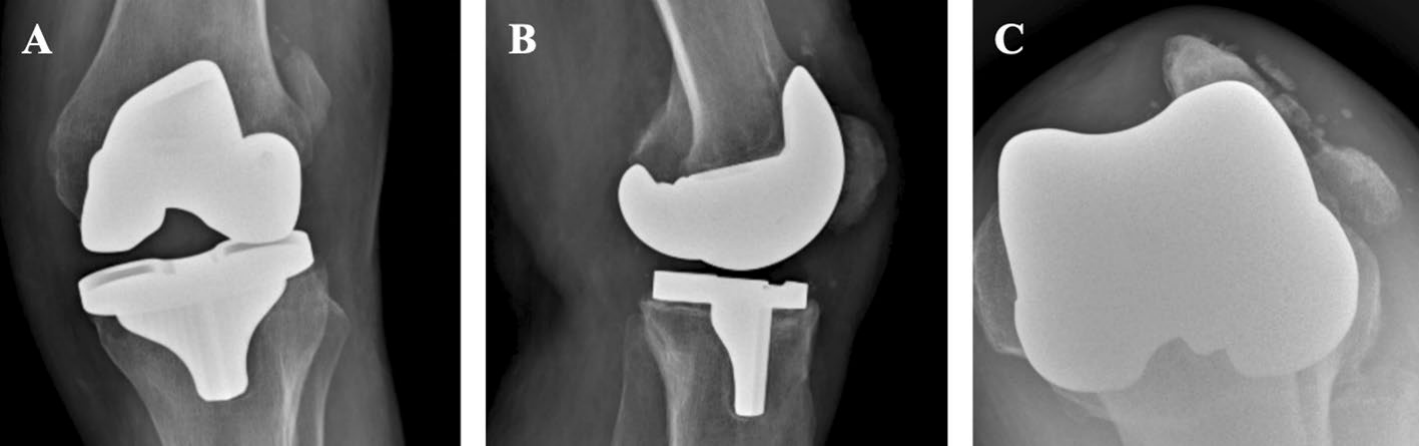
Periprosthetic patellar fracture in the non-resurfaced setting with failure of the extensor apparatus in a left knee. A Anteroposterior X-ray view; B Lateral X-ray view; C Merchant X-ray view
